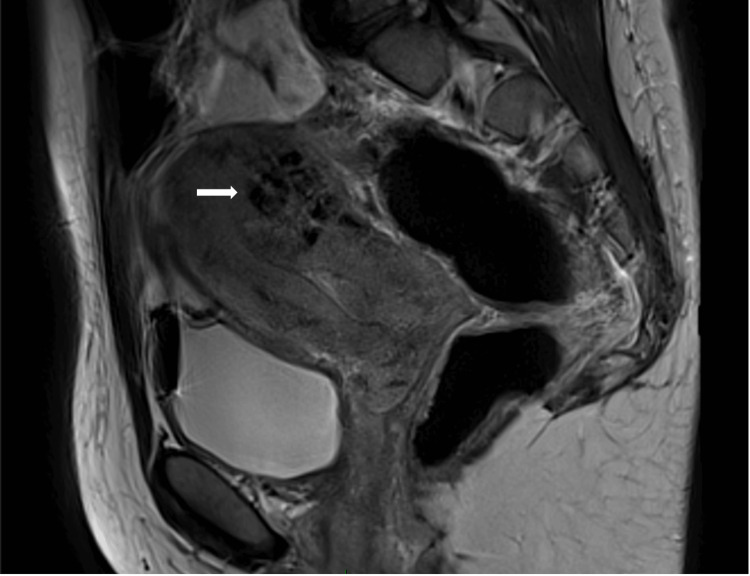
Sagittal T2-weighted sequence image showing multiple serpentine-like pathways with signal void suggestive of aberrant vessels in the posterior wall of the uterine body, with areas of interposed myometrium (white arrow) visualized.
